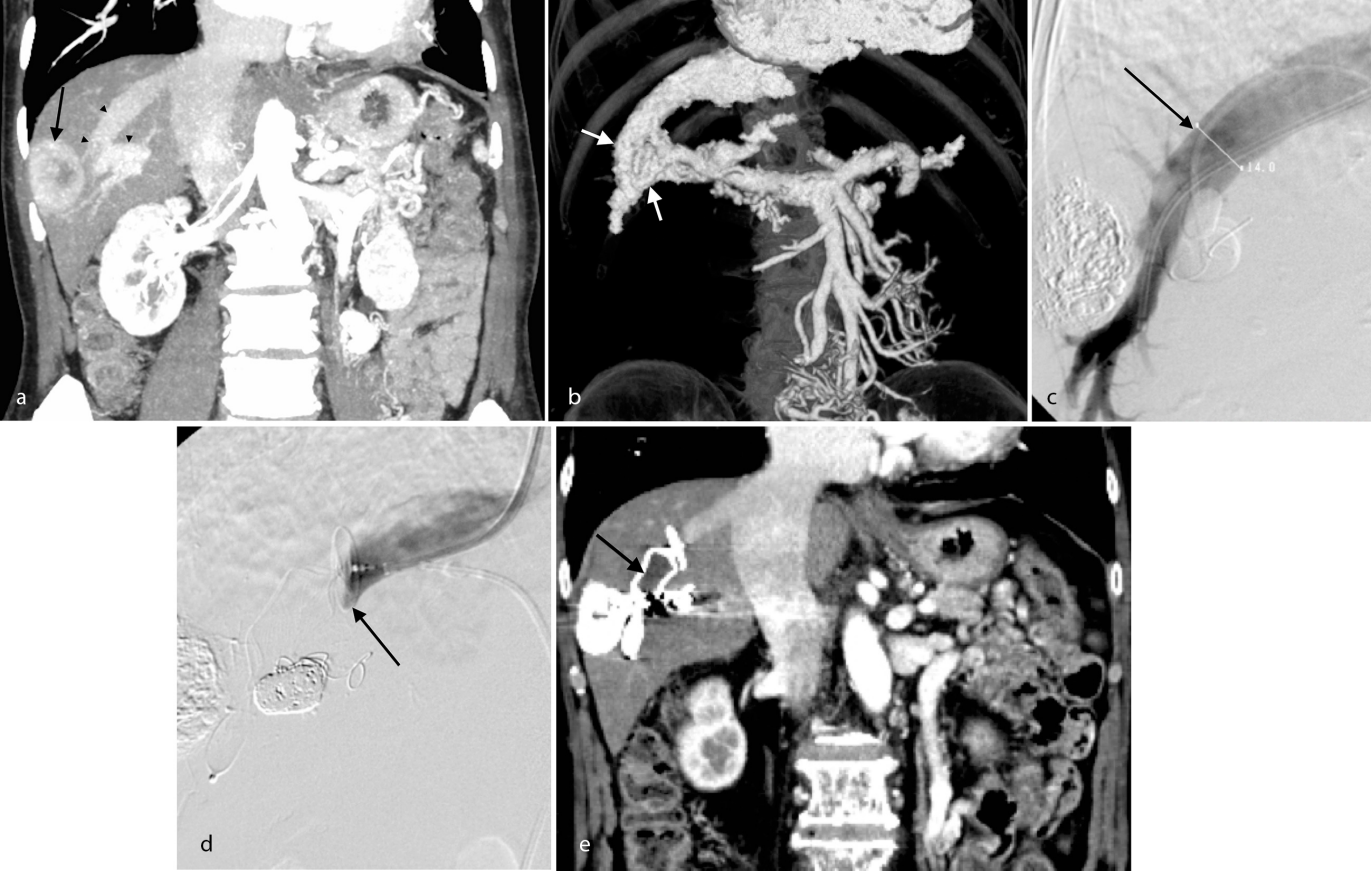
An 83 year-old male followed up for chronic hepatitis. (a) Coronal image of dynamic CT before chemoembolisation shows enhancing tumour (arrow) and a portosystemic shunt (arrowheads). (b) Volume rendering image based on abdominal dynamic CT showed a torturous shunt between the portal vein (P5) and the right hepatic vein (arrows). (c) Venography after balloon occlusion of one pathway of the portal vein hepatic vein shunt. The diameter of the right hepatic vein is 14  mm (arrow). The shunt was embolized using the Amplatzer Venous Plug II. (d) Venography after deploying the Amplatzer Venous Plug II; embolisation of the right hepatic vein is indicated (arrow). (e) Dynamic CT image 1 month after the procedure shows complete embolisation of the portal vein hepatic vein shunt (arrow).
